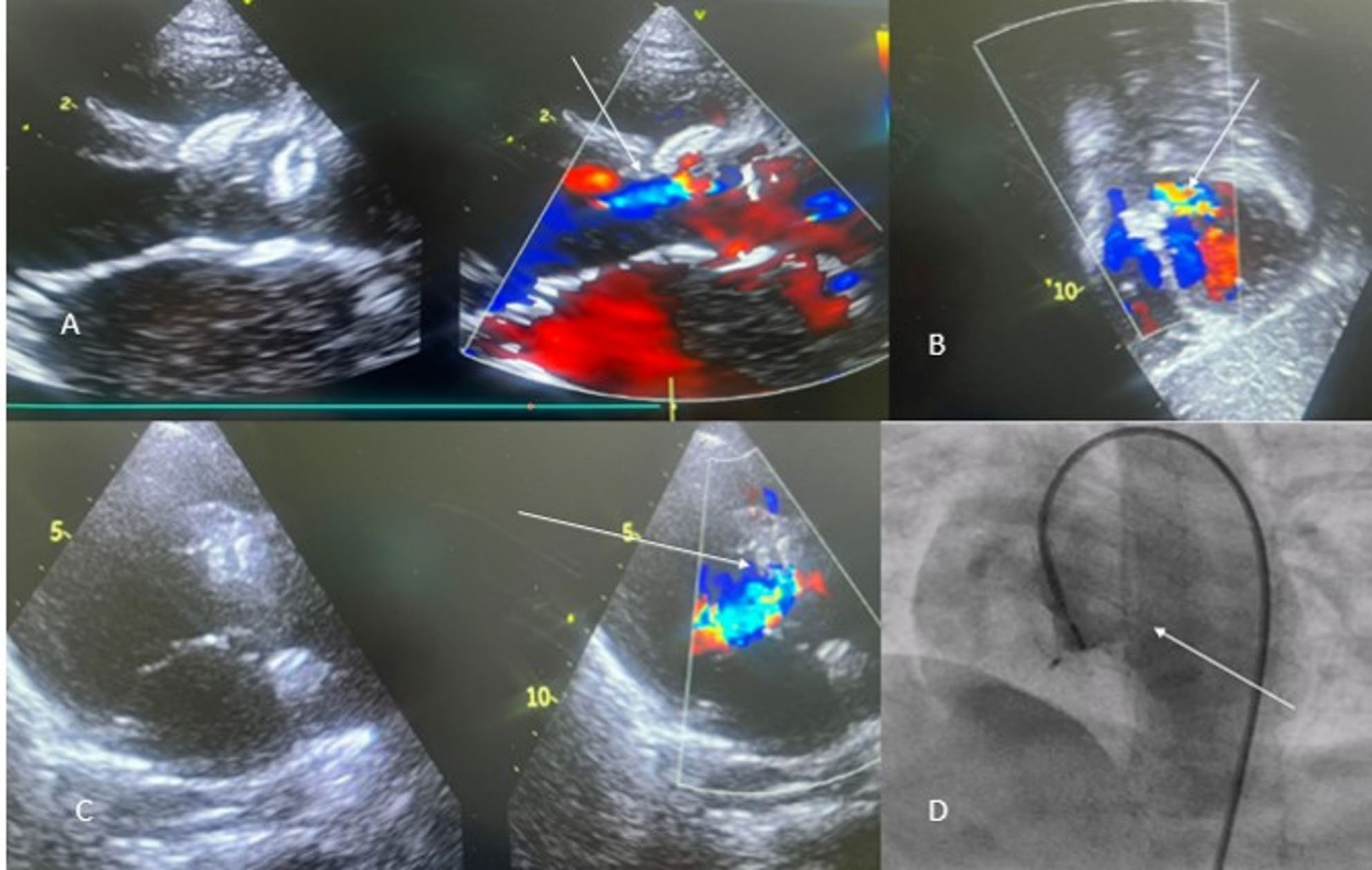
A-C arrow showing worsening of AR in failure cases after the deployment of the device in echocardiography, D depicting an angiogram showing significant AR after device placement (In all cases device was captured and taken out)
